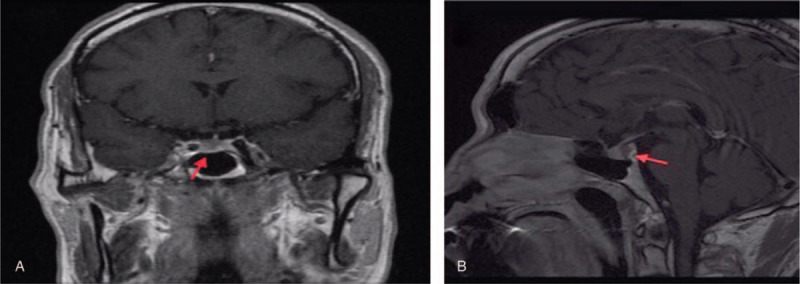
(A, B) Pituitary MRI. An abnormal signal about 0.7 × 0.5 cm was found at the pituitary. The signal intensity was slightly high on T1WI and T2WI. No clear enhancement was found on the enhanced scan. MRI = magnetic resonance imaging; T1WI = weighted imaging; T2WI = T2 weighted imaging.
